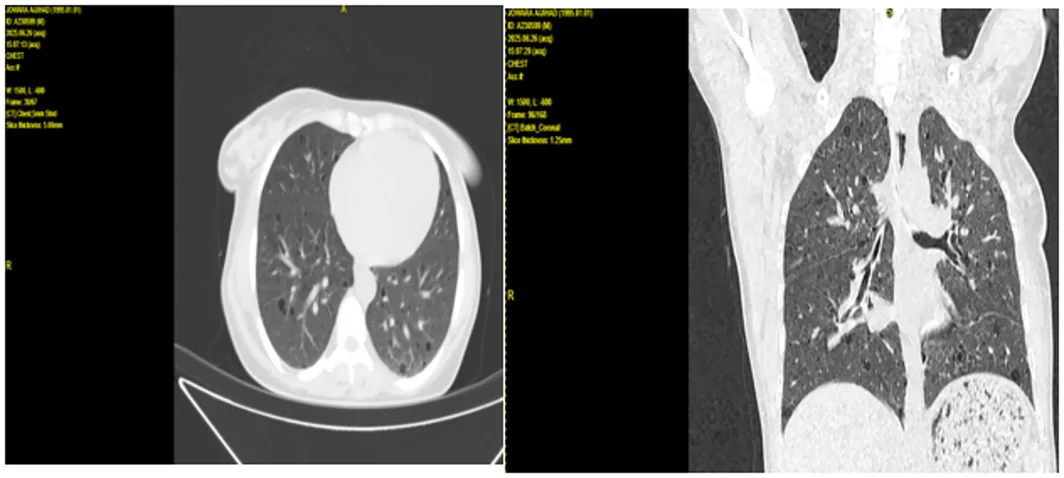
HRCT of the chest reveals bilateral thin‐walled, round lung cysts in the upper, middle, and lower lobes, indicated by red arrows. The conclusion suggests these findings are likely indicative of Lymphangioleiomyomatosis (LAM).
